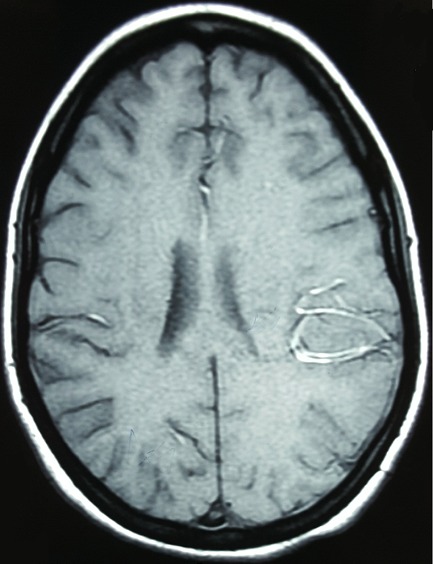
Magnetic resonance imaging. Gadolinium-enhanced axial T1 image showing a meningeal enhancement at the left parietal lobe
